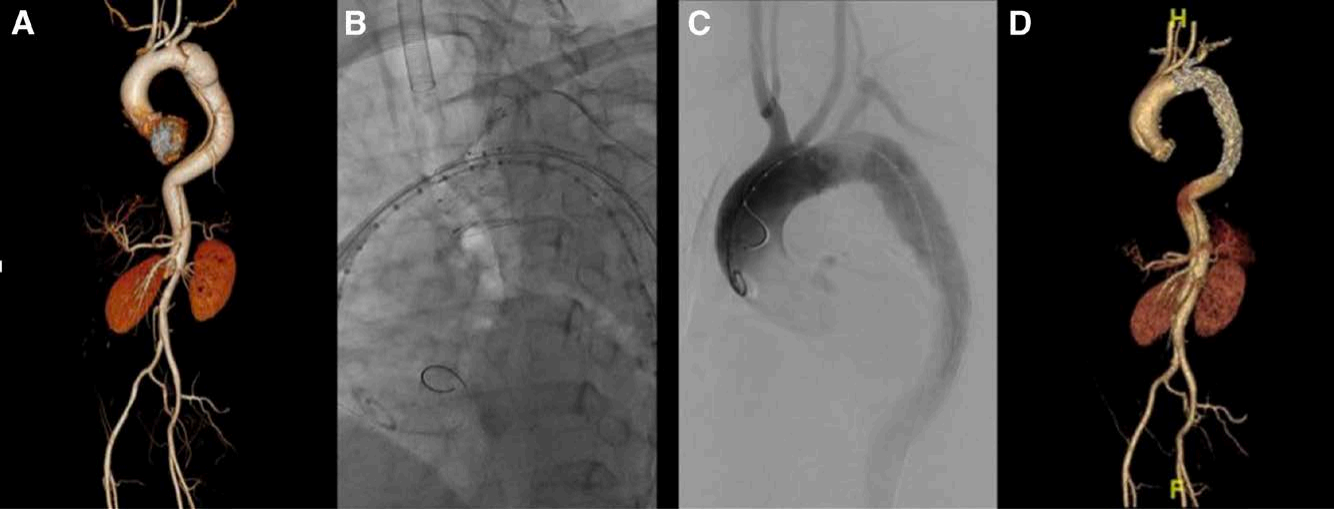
(A) CTA showed the dissection was close to the LSA and involved the thoracoabdominal aorta. (B, C) angiography showed satisfactory stent release without internal fistula. (D) Follow-up CTA showed that the stent was in good position without internal fistula and the branches were well developed. CTA = computed tomography angiography, LSA = left subclavian artery.
